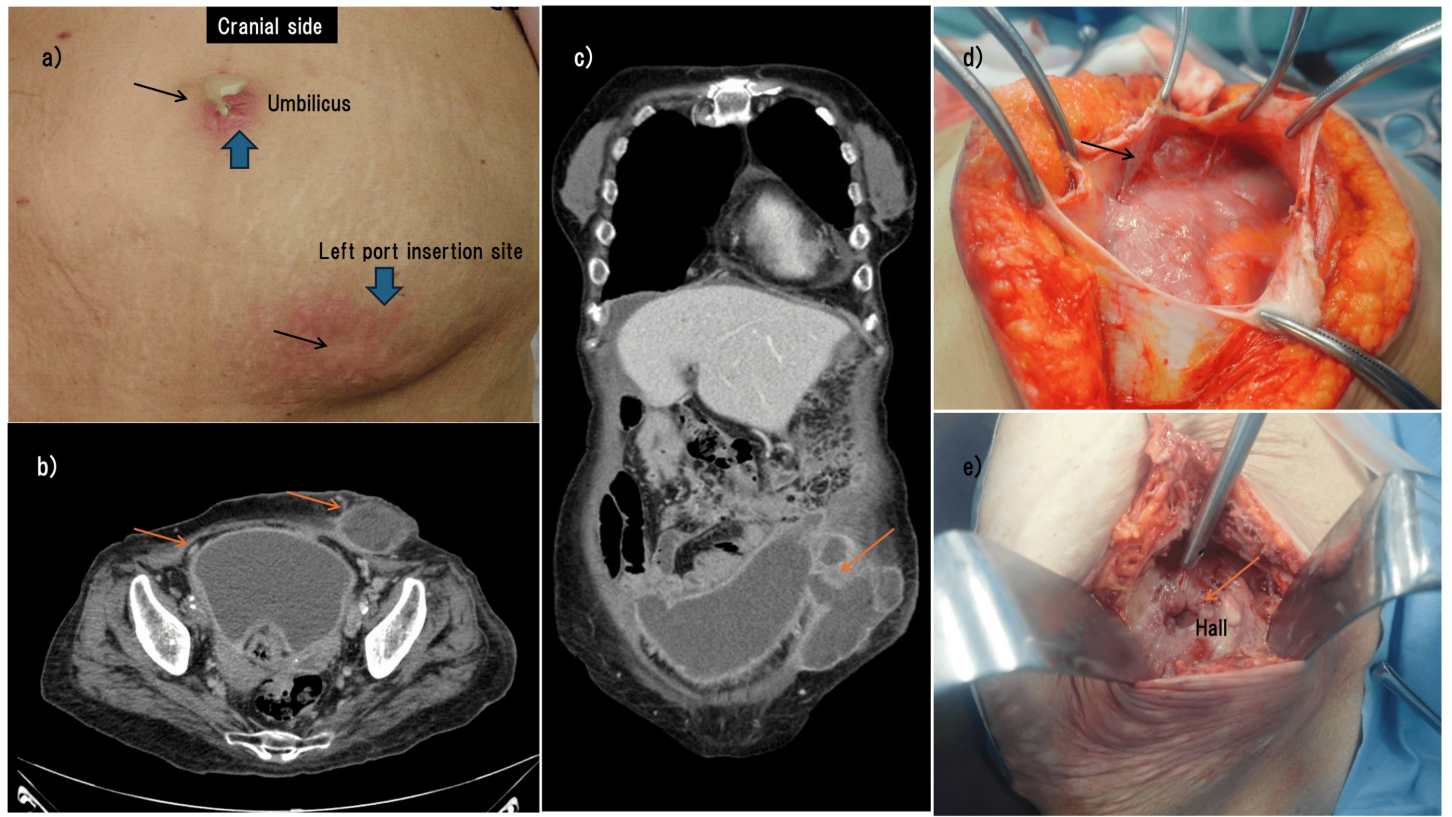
Clinical findings of the patient with abdominal abscess (a) Abscess was flowing from the umbilicus (arrow) or the laparoscopic port insertion site (arrow) in the lower abdomen; (b) The CT scan revealed an irregularly shaped subcutaneous abscess extending pelvic cavity (arrows), measuring 15×20×12 centimeters; (c) The subcutaneous abscess communicates with the pelvic abscess (arrow); (d) Massive bowel adhesion surrounding the abscess (arrow); (e) Opening the subcutaneous abscess and a hall reached the pelvic cavity was shown (arrow).
